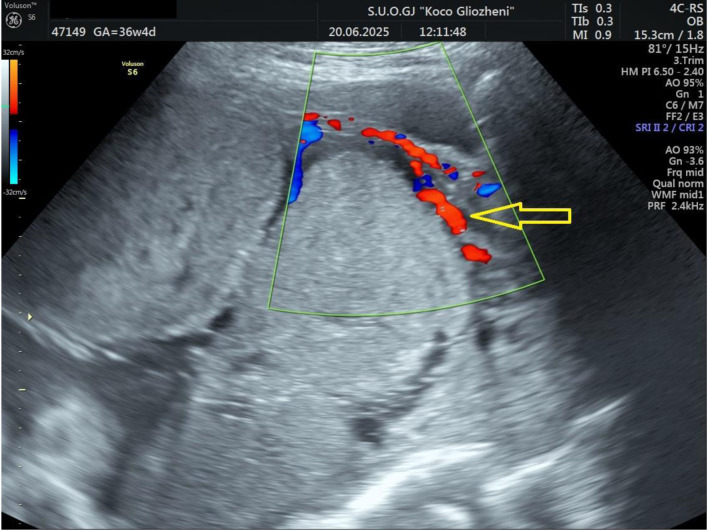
Doppler ultrasound examination showing placental tissue invading the myometrium and extending to the serosa. Increased vascular flow was demonstrated on color Doppler ultrasound (arrow).
